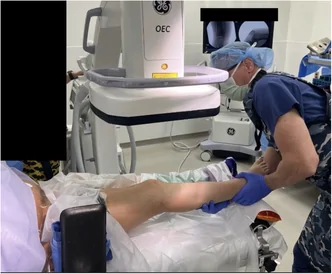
Patient positioning. Right knee, proximal to the left, distal to the right. The patient is placed supine on the operative table with a high thigh tourniquet, lateral hip post, and foot bump. If preferred, a pediatric bone foam can be placed in place of the foot bump to allow for resting 35° of knee flexion. A full‐size fluoroscopic unit enters from the contralateral or left side of the patient to obtain preoperative AP and lateral radiographs. (AP, anteroposterior.)
